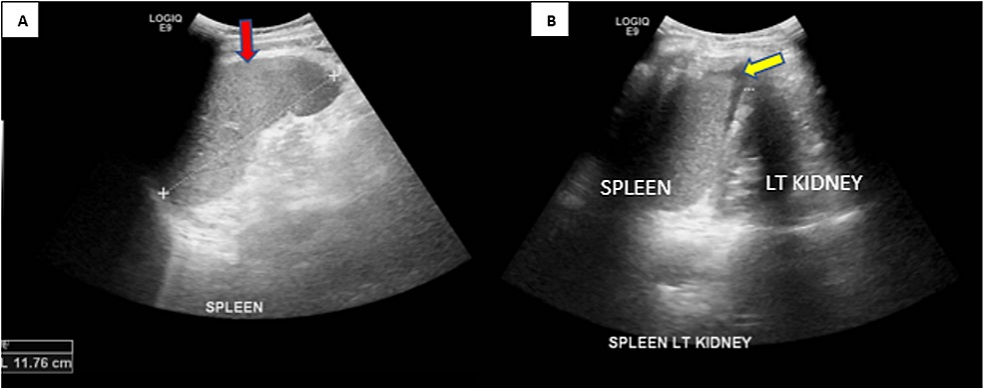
Ultrasound abdomen showing A) mildly enlarged spleen measuring 11.76 cm (red arrow) and B) trace of fluid in the splenorenal space (yellow arrow)
